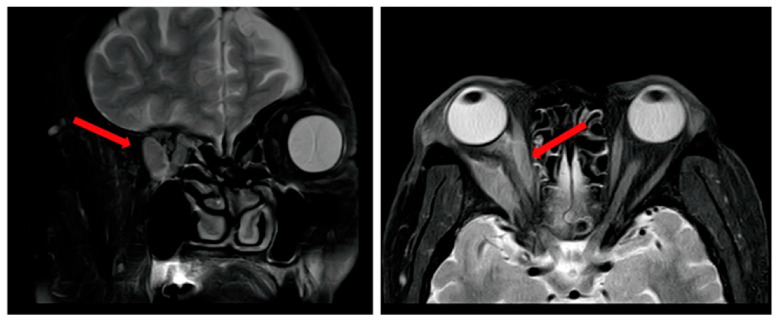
Orbital MRI demonstrated multiple myositis of the extraocular muscles, compressive displacement of the optic nerve, and right periorbital edema. The red arrow represents the compression and displacement of the optic nerve caused by the occupying effect caused by multiple extraocular myositis.
